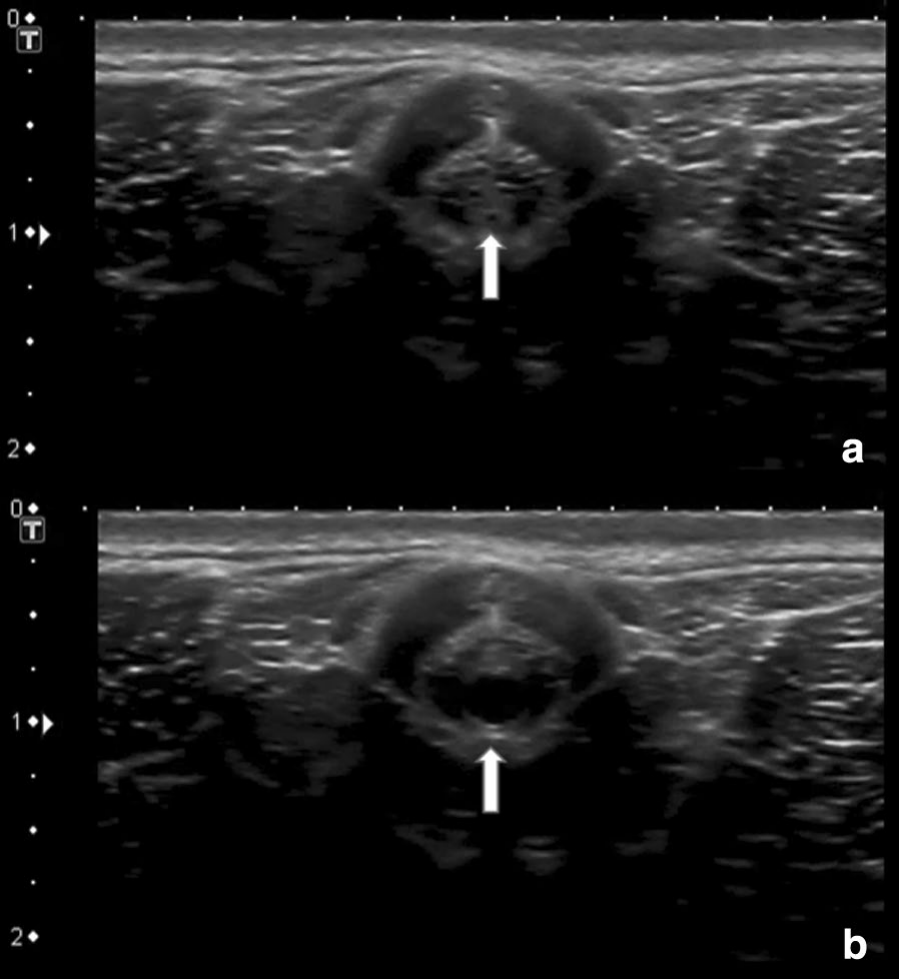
Transverse sections of the urethra during voiding. The urethra is maximally distended during voiding and collapses after suspending of the urinary outflow. a Transverse sonographic view of a collapsed urethra in a 4-week-old ram; b maximally dilated urethra of the same ram during voiding. *Arrow*: urethra
